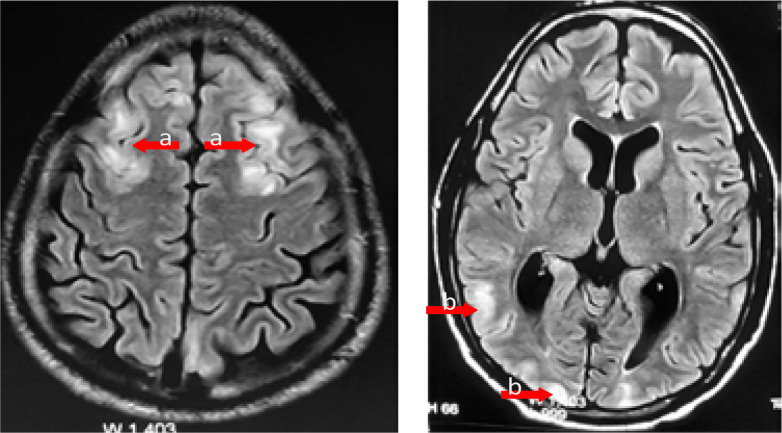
Initial T2-flair MRI showing hypersignal cerebral lesions (arrows) predominantly in the white matter of the frontal (a) and the occipital lobes (b).
